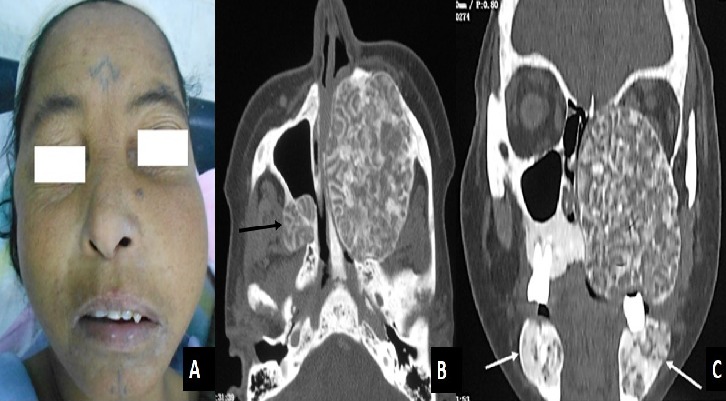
(A) swelling in the left cheek and the infraorbital region; (B ) axial CT scan image shows a destructive lesion of the left maxillary sinus with involvement associated to a lesion of the posterior wall of the right maxillary sinus (arrow); (C) coronal CT scan image shows an extensive involvement of the left maxilla and maxillary sinus associated to mandibular tumors (arrow)
